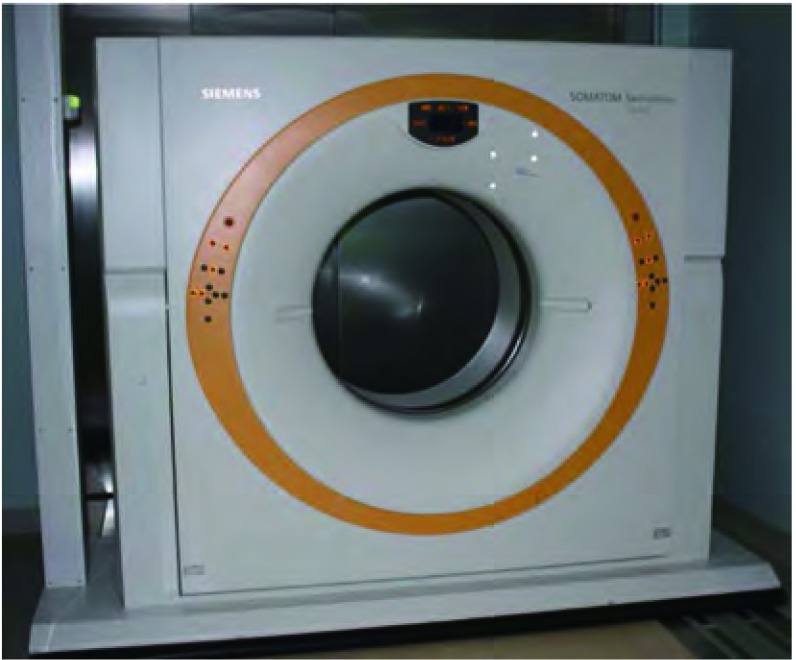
手术室中电子计算机断层扫描（CT）设备 Computed tomography (CT) scanner
